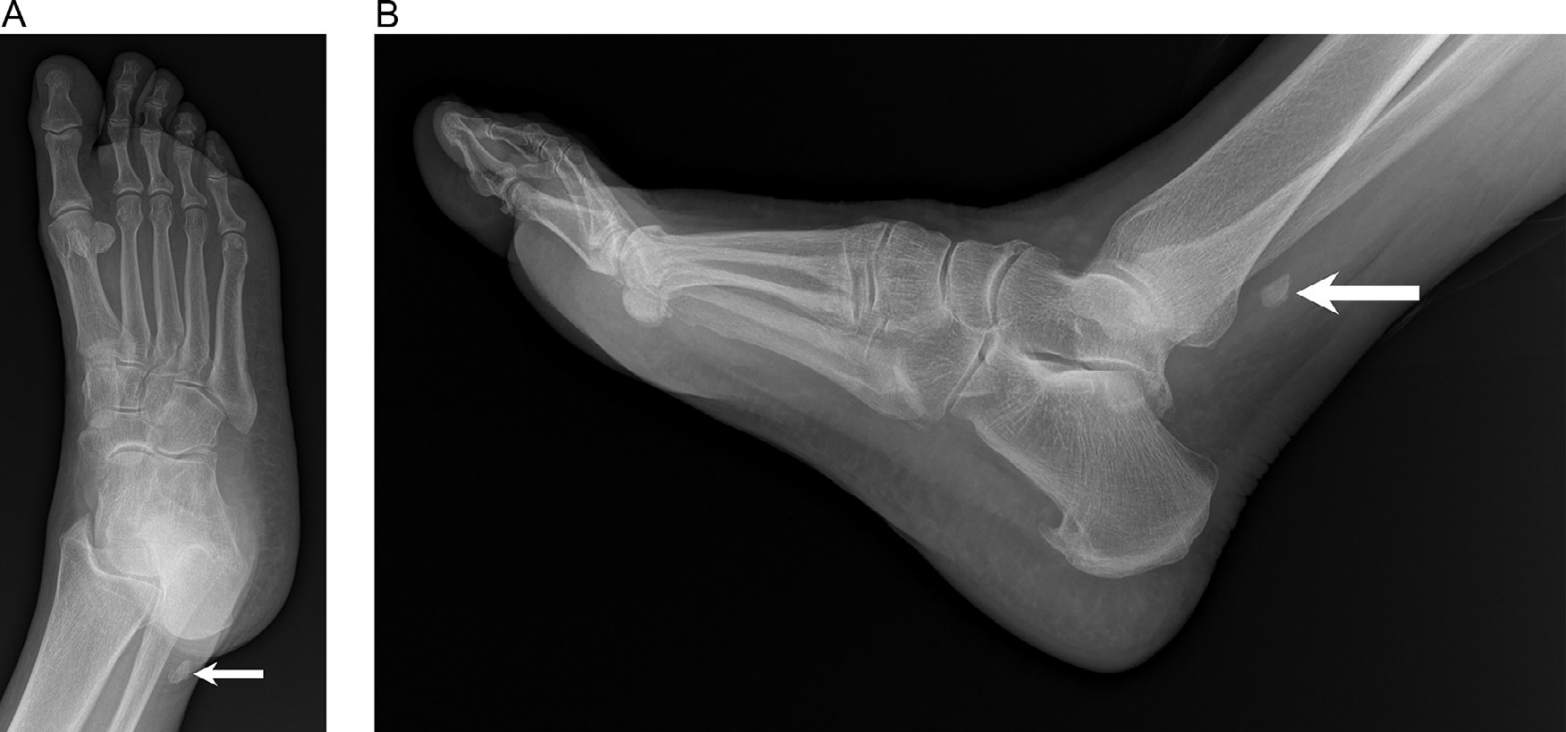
(A) Oblique and (B) lateral radiographs of the right foot 7 months after initial presentation demonstrate further proximal migration of the os peroneum, which is now located posterior to the ankle at the level of distal fibula (arrow). This suggests injury to both the IPR and SPR.
